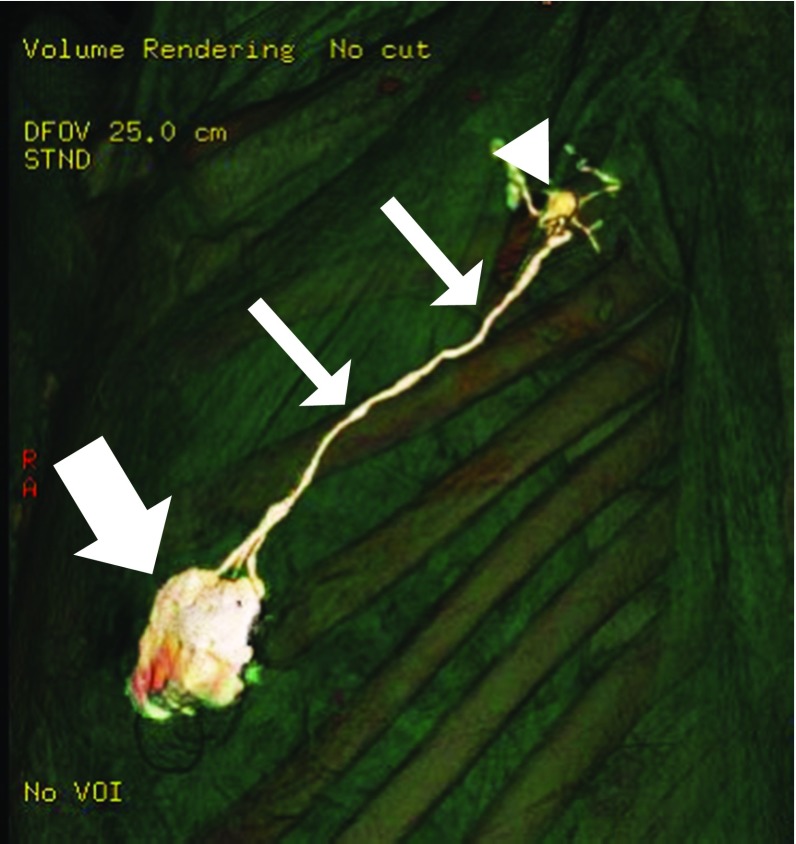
3D-CT lymphogram of woman in her 50s with left breast cancer. A single lymphatic route (thin arrow) from the periareolar area (thick arrow) drains into a single SLN (arrowhead)
